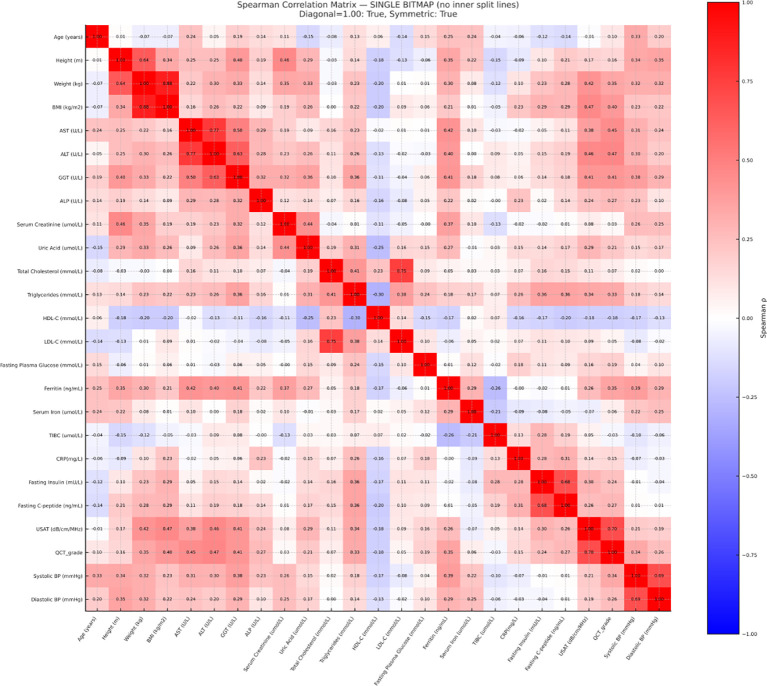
Spearman correlation matrix between USAT and metabolic, hepatic, and anthropometric parameters. The heatmap depicts the pairwise Spearman’s rank correlation coefficients (ρ) among the ultrasound attenuation (USAT) value and multiple clinical, biochemical, and anthropometric parameters in the study cohort. Color intensity reflects the strength and direction of the correlation (red: positive; blue: negative), with exact ρ values displayed in each cell. Variables include liver enzymes (ALT, AST, GGT, ALP), lipid profile (Triglycerides, HDL-C, LDL-C, Total Cholesterol), glycemic indices (Fasting Plasma Glucose, Fasting Insulin, Fasting C-peptide), iron metabolism markers (Ferritin, Serum Iron, TIBC), renal function parameters (Serum Creatinine, Uric Acid), inflammatory marker (CRP), and anthropometric measures (BMI, weight, height, waist-to-hip ratio, blood pressure).
